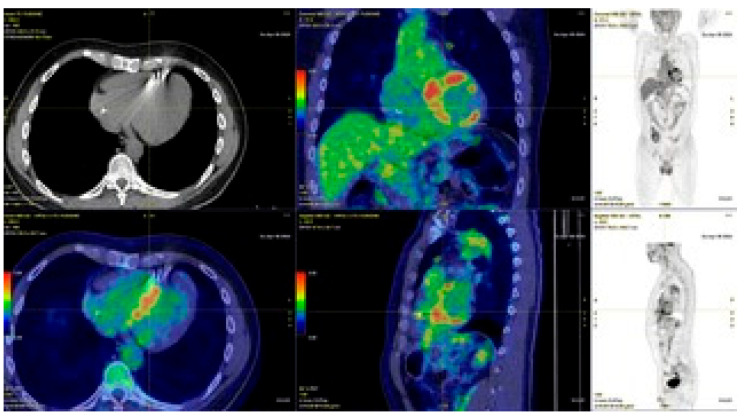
PET-TC with FDG showing disomogeneous intense myocardial uptake, involving the interventricular septum and inferior wall.
